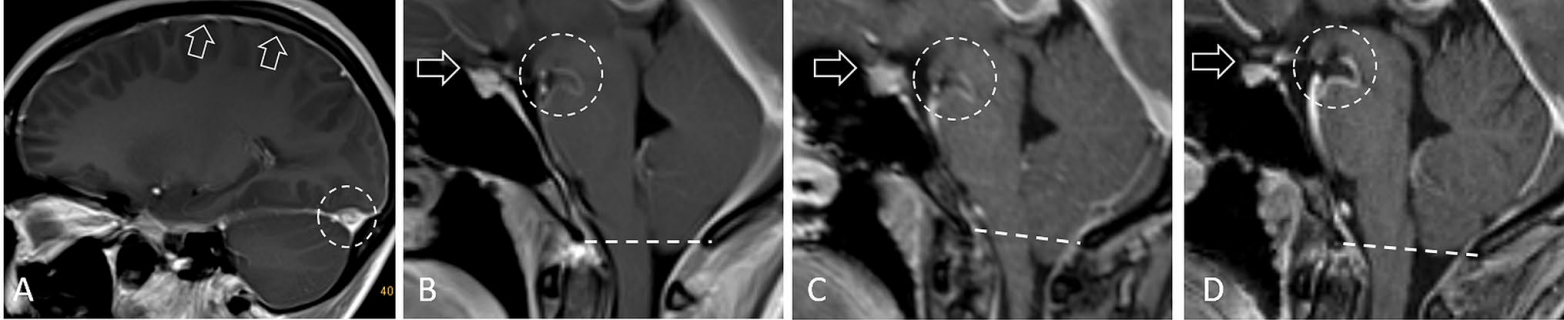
Imaging signs on MRI of the head. First MRI (see also timeline, supplementary file 1) showing typical intracranial signs of spontaneous intracranial hypotension (SIH) with pachymeningeal enhancement (open arrows in A) and engorgement of transverse sinus (dashed-line circle in A) as well as narrowing of the suprasellar (open arrow in B) and mamillopontine distance (dashed-line circle in B). Sagging of the cerebellar tonsil is only slightly visible (dashed-line in B). Second MRI is unchanged (not shown). At third MRI 11 months after onset of symptoms, pachymeningeal enhancement and venous engorgement disappeared (not shown), but sagging of the cerebellar tonsil increases (dashed-line in C). At the fourth MRI 3 months after surgical ligation of the CSF-lymphatic fistula, suprasellar and mamillopontine distances, and tonsil herniation normalized (D)
